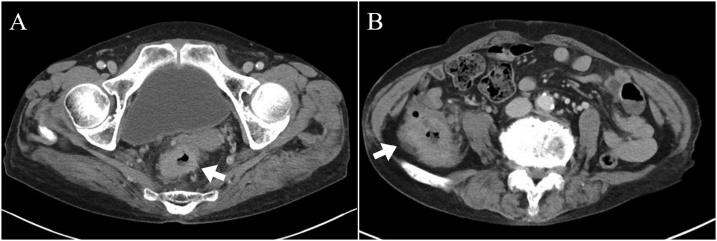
Computed tomography (CT). CT shows a mass in the rectum (A) and ascending colon (B).
